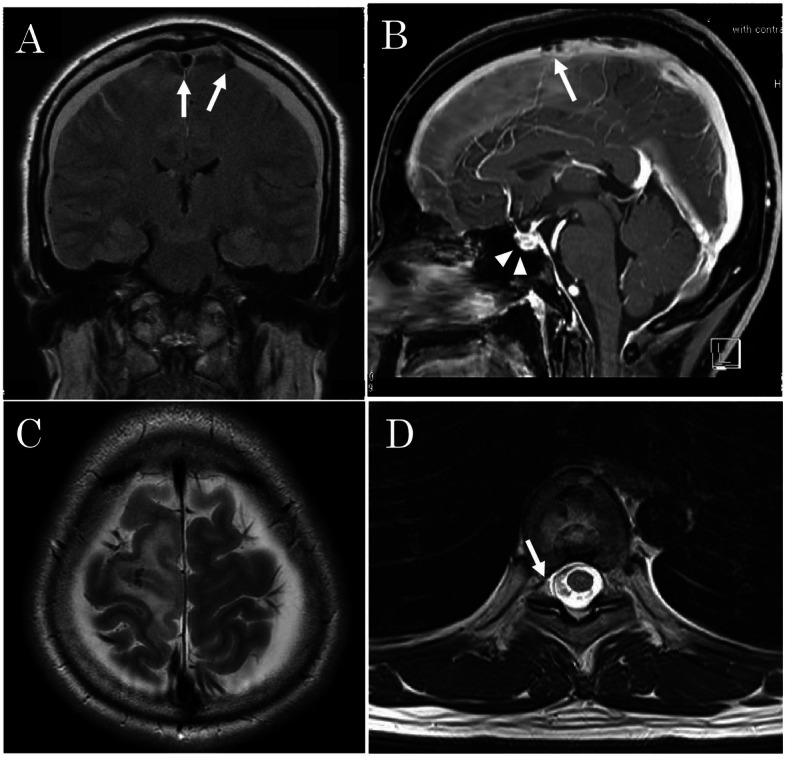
a Coronal T1-weighted gadolinium-enhanced MRI showing diffuse pachymeningeal enhancement and cortical vein thrombosis (arrows). b Sagittal T1-weighted MRI showing enlargement of the pituitary gland (arrow head) and cortical vein thrombosis (arrow). c Axial cranial MRI showing cerebral edema. d Spinal MRI showing anterior epidural fluid collection at the level of the T4–T10 spinal canal (arrow). MRI, magnetic resonance imaging
